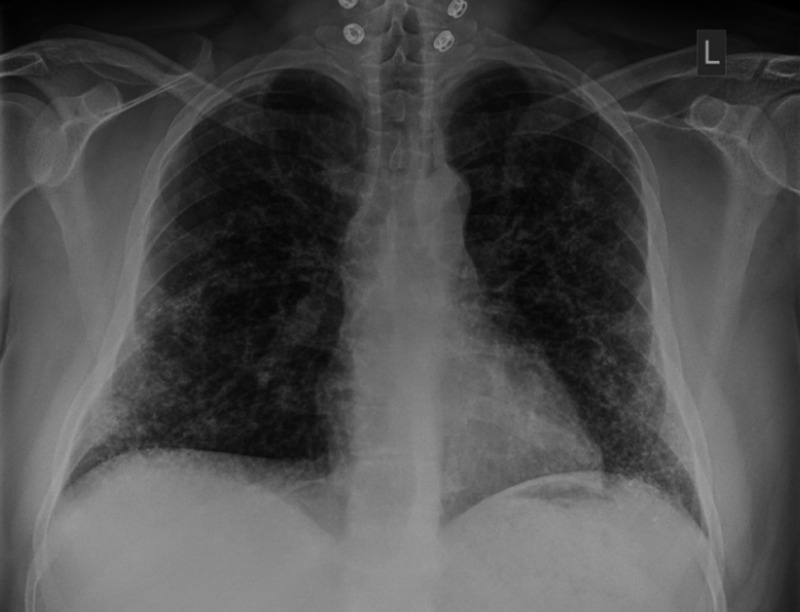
Erect chest X-ray (posteroanterior view) In this chest X-ray, we can see numerous tiny dense nodules extensively distributed in both lungs especially in the lower zones and in a branching (dendriform) pattern. Reticulations from thickening of the interlobular septa can be appreciated as well.
